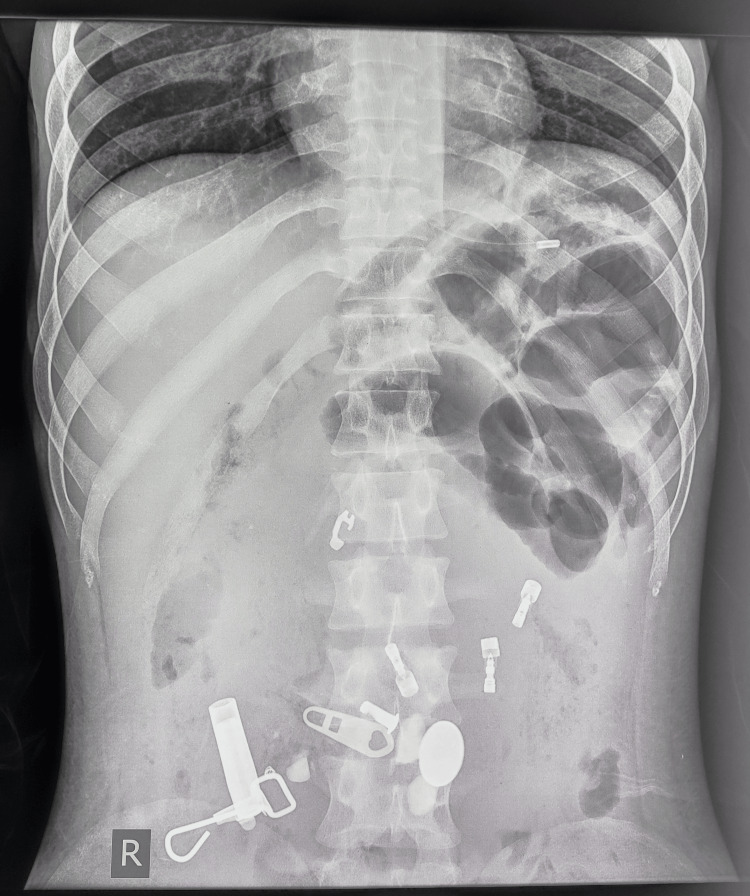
X-ray of the abdomen showing foreign bodies in the gastrointestinal tract
